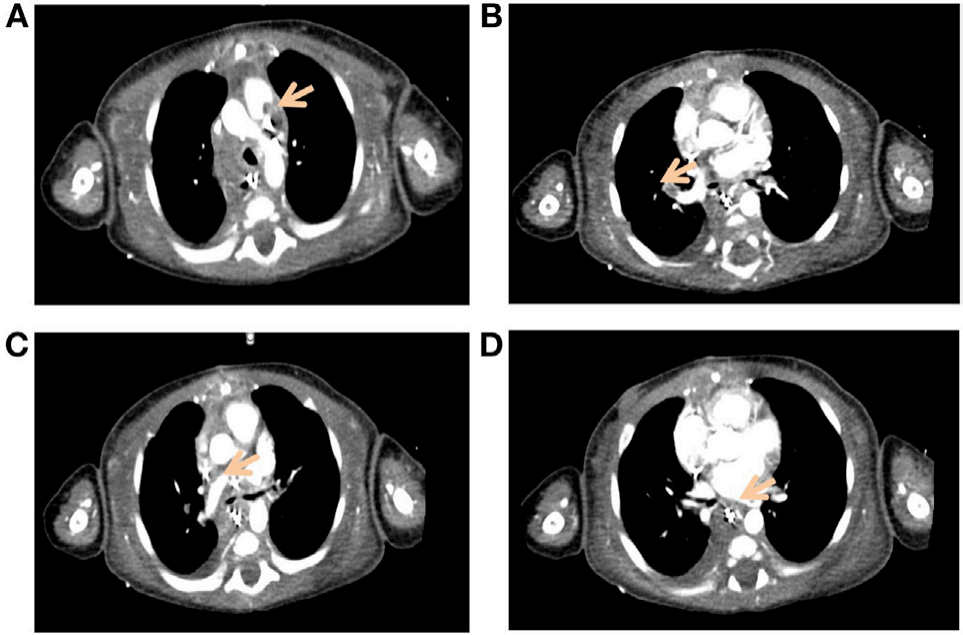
Axial view of the pulmonary artery on angio-CT of the chest. The images show bilateral pulmonary artery thrombosis (arrows), with thromboses of the main pulmonary artery (A), of the upper branch of the right pulmonary artery (B), and of the upper (C) and lower branches of the left pulmonary artery (D).
